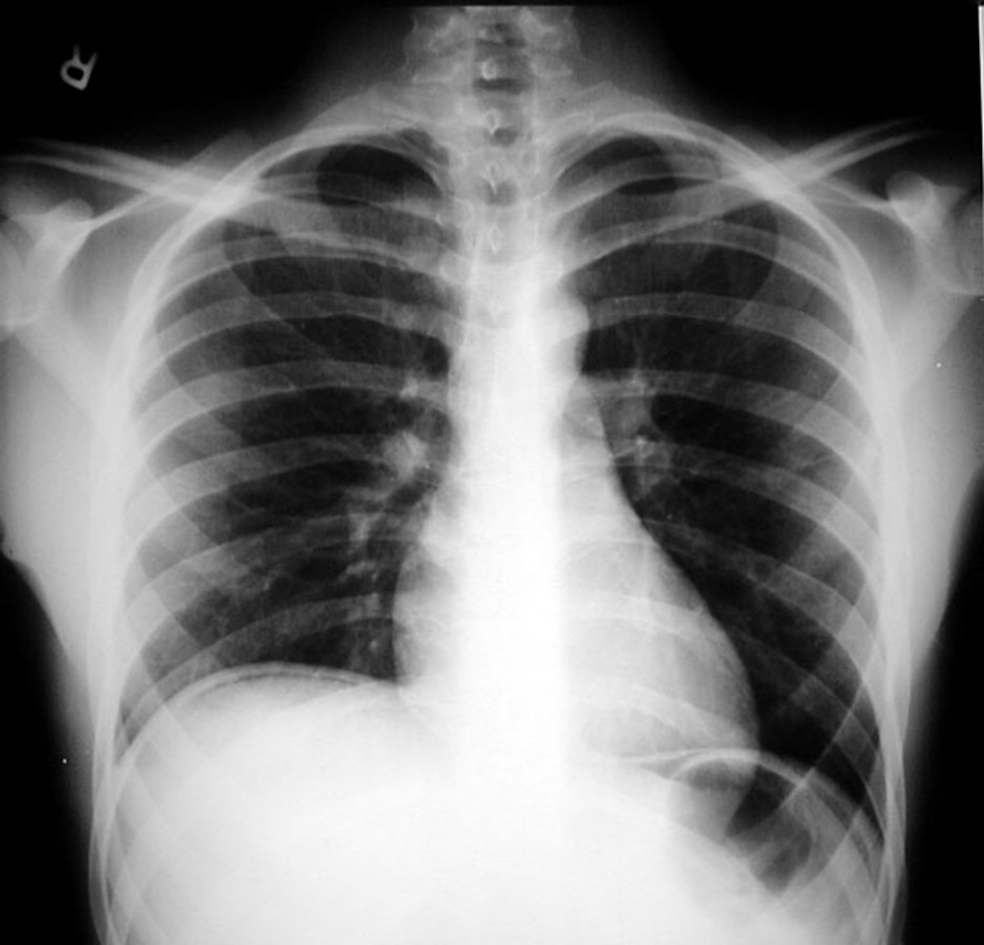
X-ray chest showing free gas under right hemidiaphragm.
